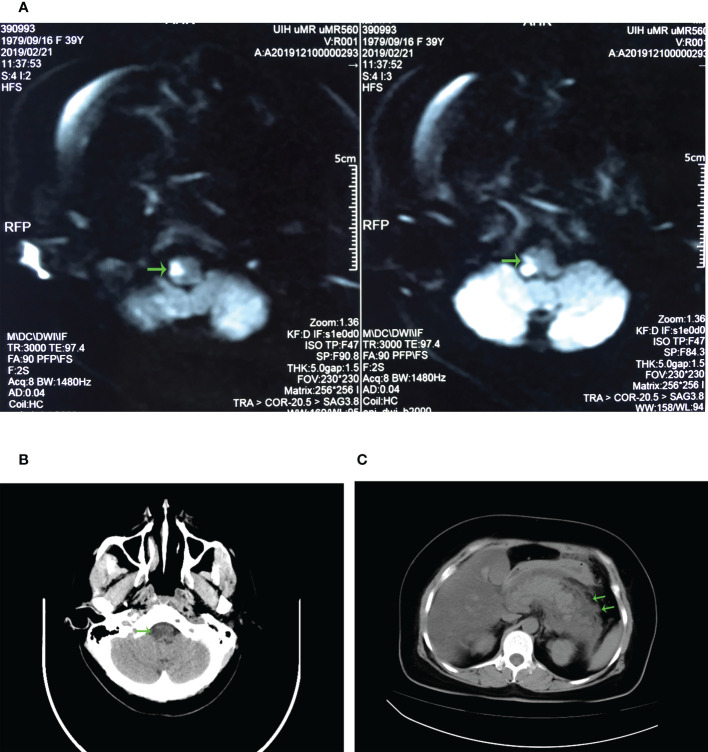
Radiology results. (A) Patient’s cranial DWI imaging. The green arrow marks the abnormal signal on the right side of the patient’s medulla oblongata, which is considered as acute cerebral infarction. (B) CT imaging of the patient’s head. The green arrow marks a small hypodense lesion on the right side of the medulla oblongata, suggesting the possibility of infarction on the right side of the medulla oblongata. (C) CT imaging of the patient’s abdomen. The green arrow marks the swelling and exudation of the pancreas, indicating acute pancreatitis.
